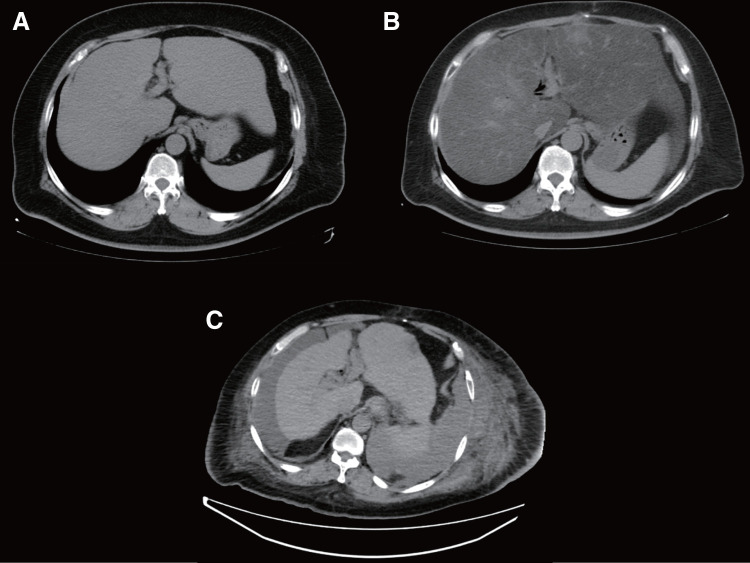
Chronologic changes in CT findings in Case 1. (A) CT before PD showed hepatic steatosis. The CT value of the liver was 40 HU. (B) CT 6 months after PD showed progression of hepatic steatosis. The CT value was −2 HU. (C) CT before liver transplantation showed an atrophic liver with an irregular surface and blunt edge, consistent with complete liver cirrhosis. The CT value increased to 40 HU, indicating progression of liver fibrosis. PD, pancreaticoduodenectomy; HU, Hounsfield units
